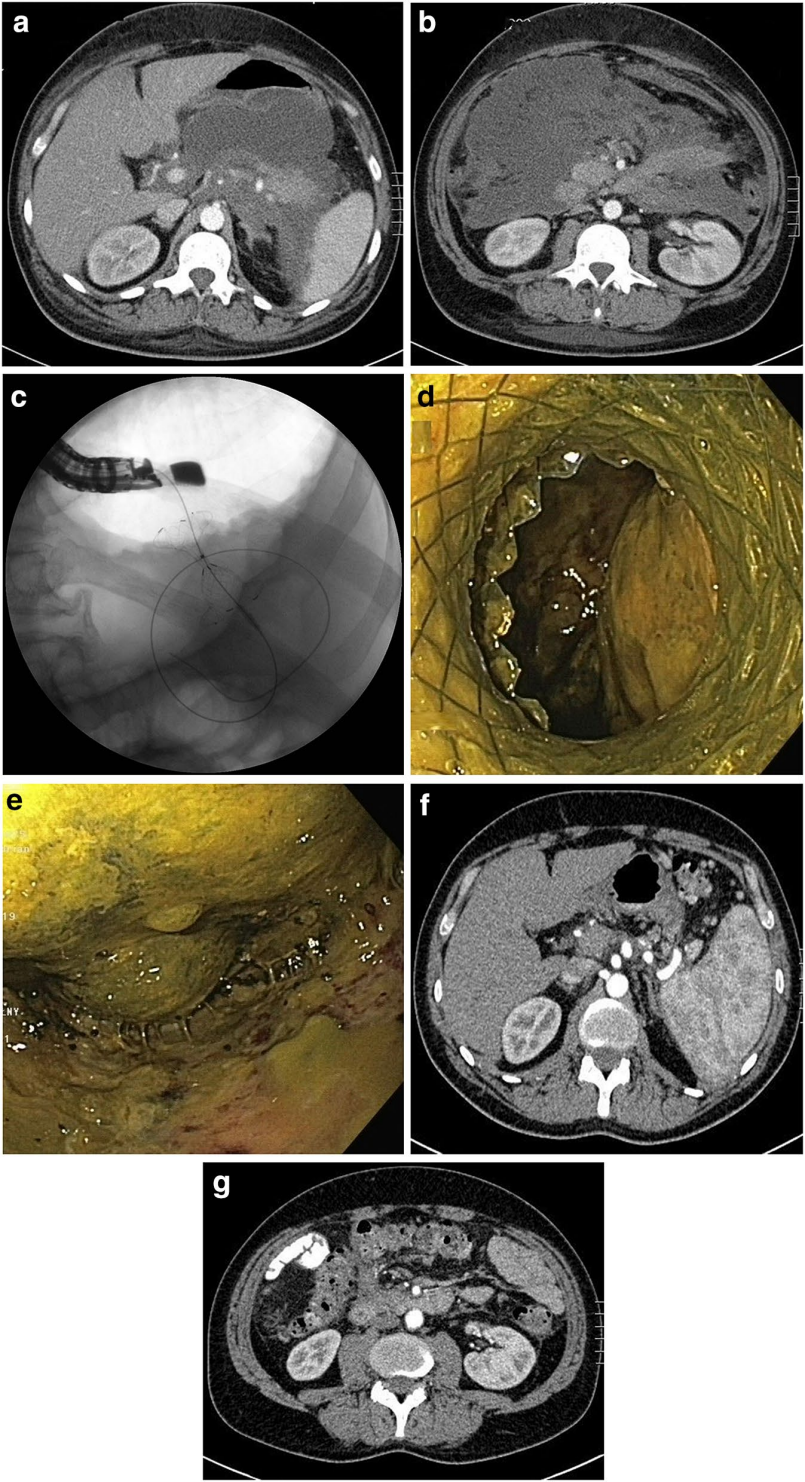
a–g Endoscopic treatment of acute necrotic collections. A 35-year-old male with acute necrotizing pancreatitis. On contrast-enhanced abdominal and pelvic computed tomography on day 8 of pancreatitis, extensive acute necrotic collections are visible (a, b). Because of symptoms of abdominal compartment syndrome, transmural endoscopic drainage of acute necrotic collections was performed. On fluoroscopy, the drainage system can be seen (c). During direct endoscopic debridement, solid necrotic masses within the lumen are visible (d, e). On control multiphase contrast-enhanced abdominal and pelvic computed tomography (f, g) after 6 months since the endoscopic treatment, complete regresion of necrotic collections was noted.
